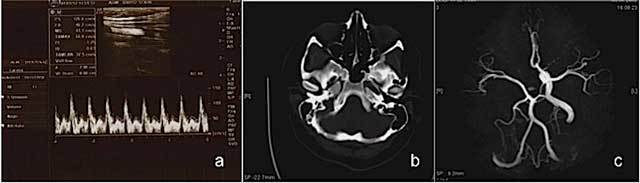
Ultrasound and Color Doppler images reveal right ICA agenesis and externalization flow pattern of right CCA (a); an axial CT image at bone window reveals absent right bony carotid canal (b); MR angiography image shows right ICA agenesis and anomalous origin of ophthalmic artery (c) in Case 1.
